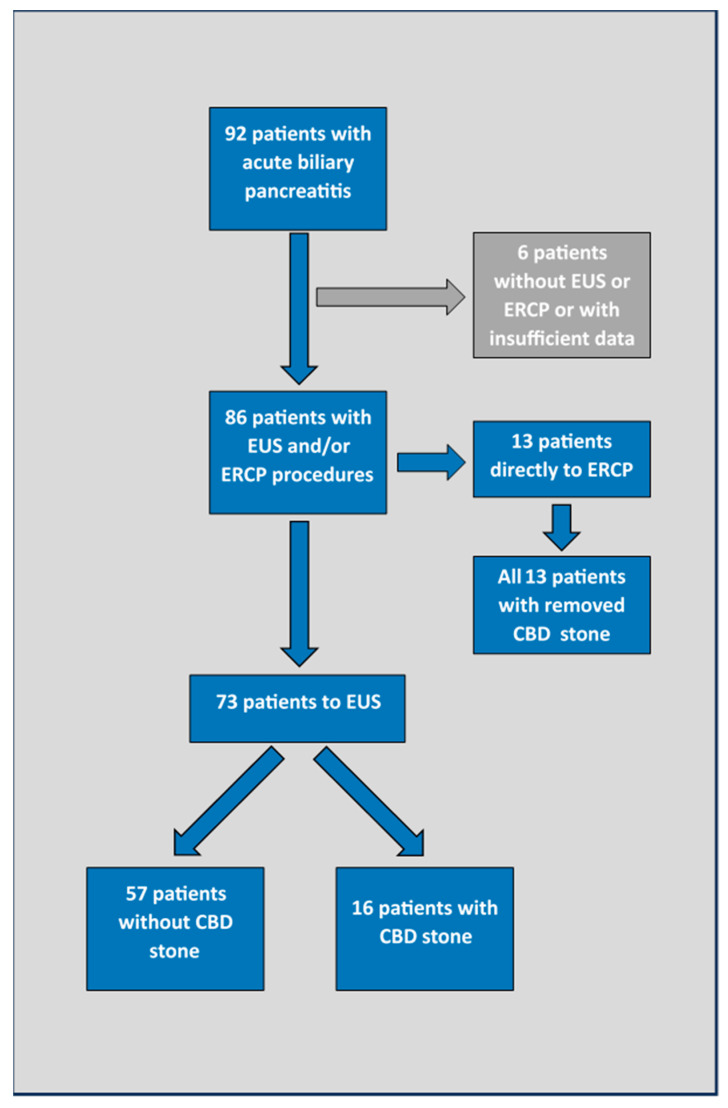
Patient flow. EUS—endoscopic ultrasound; ERCP—endoscopic retrograde cholangiopancreatography; CBD—common bile duct.
